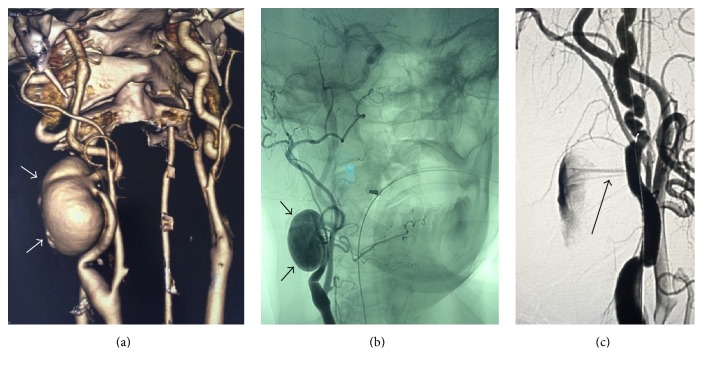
(a) Preoperative CT, the arrow points to the aneurysm; (b) intraoperative angiography, the arrow points to the aneurysm; (c) intraoperative angiography after first stent graft deployment, the arrow points to the leak.
